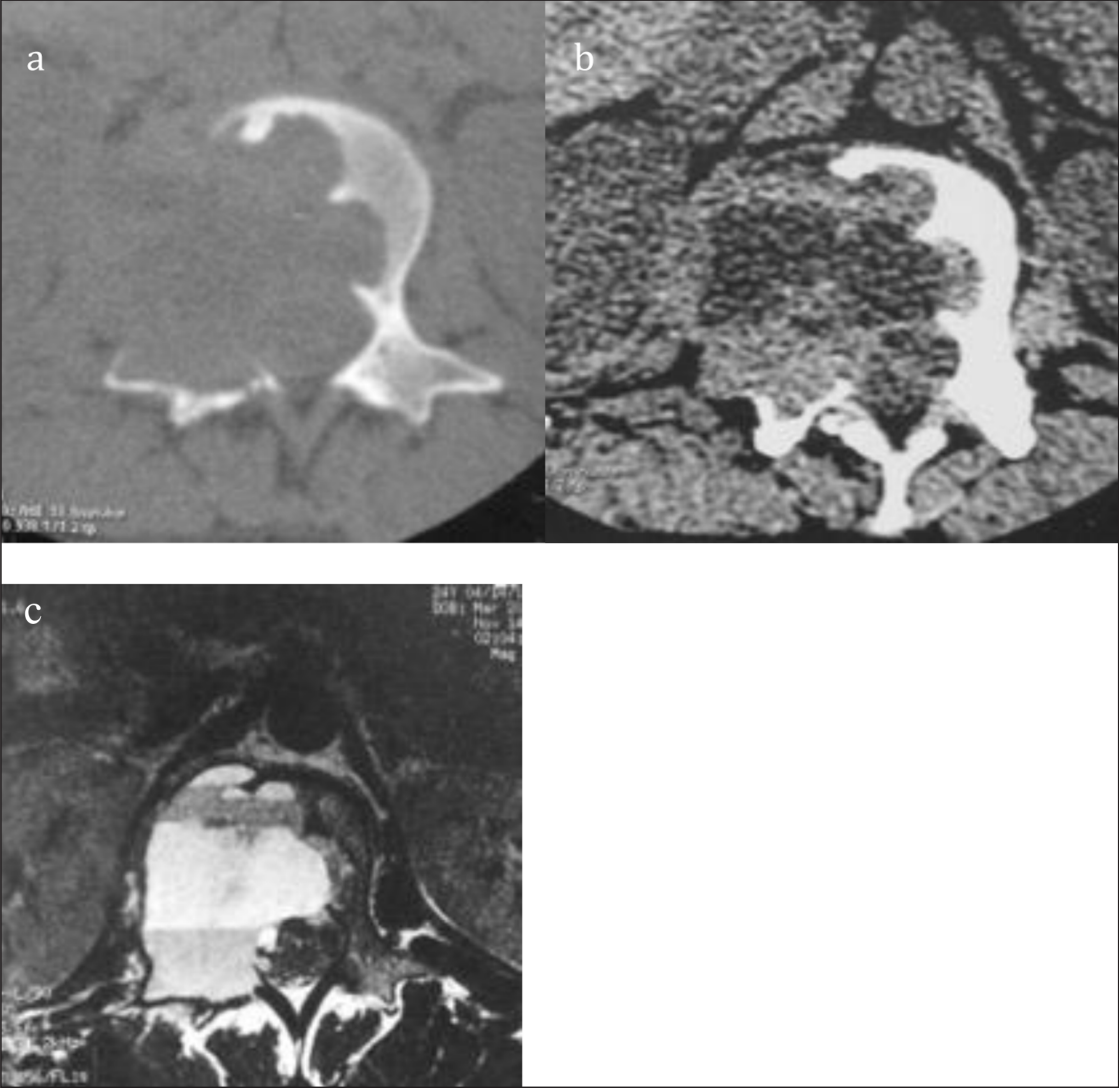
Giant Cell Tumor of L2. Axial CT scan of L2 shows a multiloculated lytic lesion (a) with intralesional fluid–fluid levels (b). Axial T2-WI MRI confirms an expansile multiloculated lesion of the body and neural arch containing fluid-fluid levels (c).
